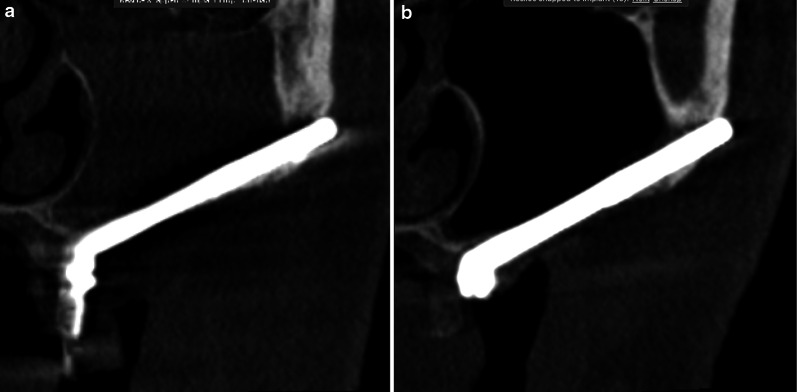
a The CBCT of patient 18 taken ten days after the surgery showed a positive M-LM score with almost complete maxillary sinus opacity and the clinical LK test was also positive. The patient was diagnosed with acute rhinosinusitis and treated with antibiotics and local corticosteroids. b The CBCT image of patient 18 taken 1 year postoperatively. Total transparency of the sinus was present; the M L-M score was negative. (In collaboration with Drs. Guy Mclellan and Ophir Fromovich.)
